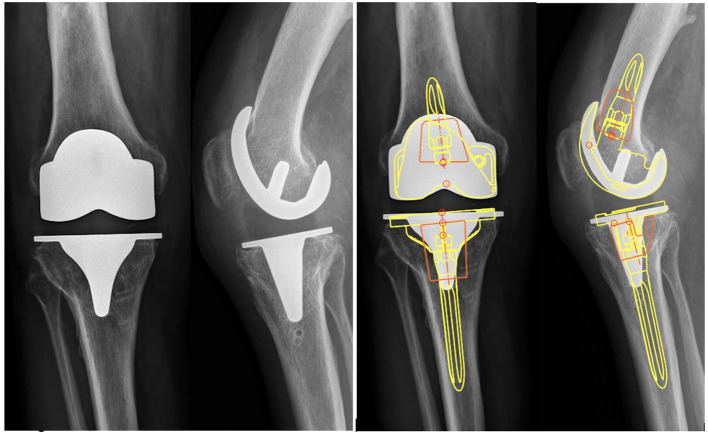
Assessment of the necessity of offset stems on the preoperative radiographs with the Traumacad^®^ software (Yellow: implants and stem; Orange: metaphyseal cones).
